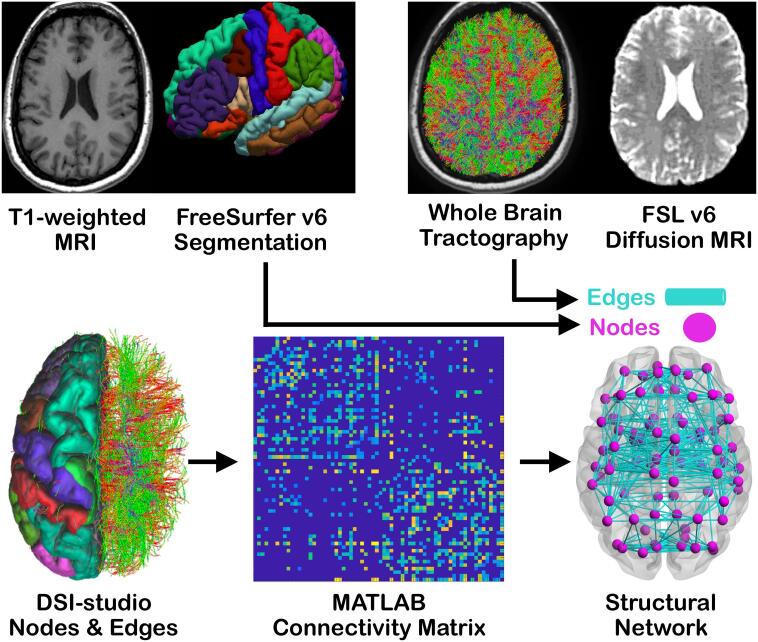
Processing steps shown on an example participant. Data was processed with FreeSurfer (T1w), FSL (dMRI), DSI-studio (combined nodes and edges), MATLAB (connectivity matrix) and NBS (structural network). As seen from left to right and top to bottom. T1w = T1-weighted MRI; dMRI = diffusion MRI; NBS = network-based statistics.
